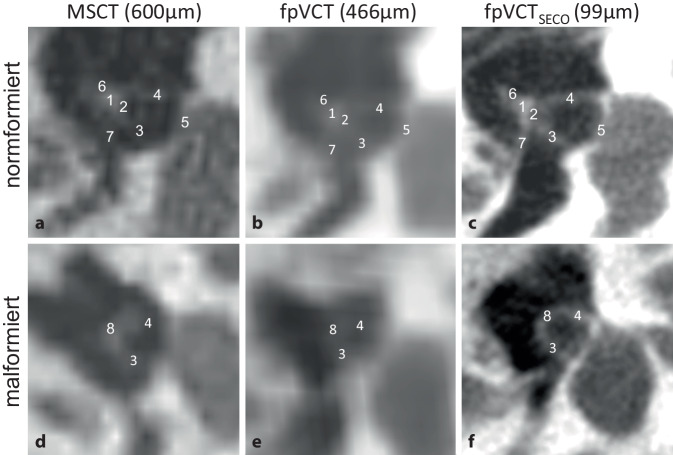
Darstellung des Stapes mit verschiedenen Bildgebungsmodalitäten und -einstellungen. Repräsentative Bilder eines normformierten Stapes mit MSCT (600 µm Schichtdicke) sind in a, fpVCT (466 µm Schichtdicke) in b und fpVCT_SECO_ (99 µm Schichtdicke) in c dargestellt. d–f zeigen die Ansicht des Stapes bei Fehlbildungen. Folgende Substrukturen des Stapes wurden identifiziert: *1* Caput stapedis, *2* Collum stapedis, *3* Crus anterius stapedis, *4* Crus posterius stapedis, *5* Basis stapedis, *6* Art. incudostapedialis, *7* M. stapedius, *8* fehlgebildeter Stapes. *MSCT* Multislice-Computertomographie, *fpVCT* „flat-panel volume computed tomography“, *fpVCT*_*SECO*_ sekundäre Rekonstruktionen der fpVCT
